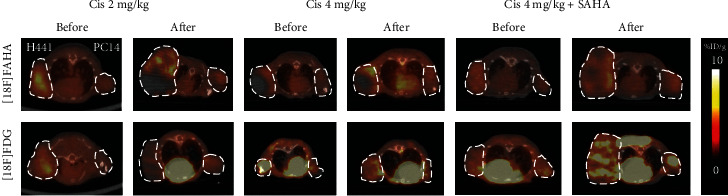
Axial tumor [^18^F]FAHA (upper panel) and [^18^F] FDG (lower panel) imaging before and after drug administration. [^18^F]FAHA PET/CT images were summed over 10 min (20–30 min after [^18^F]FAHA injection). Cisplatin significantly increased [^18^F]FAHA radioactivity in H441 and PC14 xenograft tumors, and this effect was blocked by SAHA. [^18^F] FDG imaging at 45–55 min after injection. Tumor [^18^F] FDG accumulation decreased after administration of cisplatin, but increased after administration of cisplatin with SAHA.
